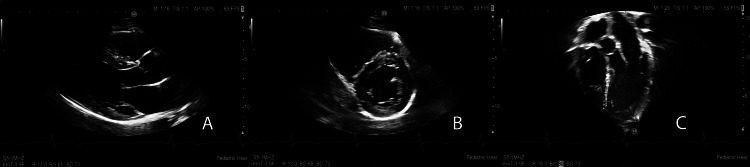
Echocardiogram after resolution of ventricular hypertrophy Transthoracic echocardiography of the parasternal long axis (A), parasternal short axis (B), and apical three-chamber view (C) were obtained 45 days after the initial presentation for MIS-C. There was no hypertrophy of the left ventricle.
